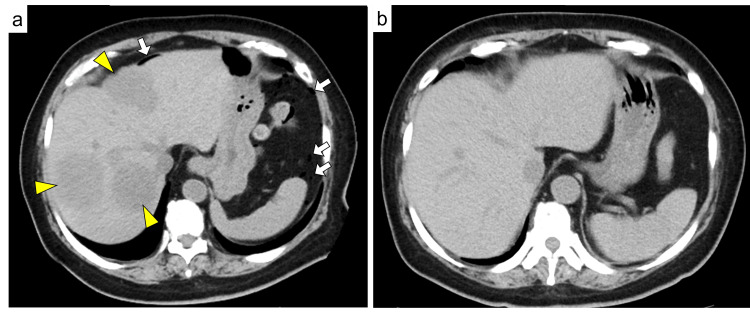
Abdominal computed tomography findings (a) Preoperative imaging revealed free air on the liver surface (arrows). The site of perforation was not identifiable. Multiple low-attenuation areas were observed in the liver (arrowheads). (b) On postoperative day 14, the low-attenuation areas in the liver had almost completely resolved.
